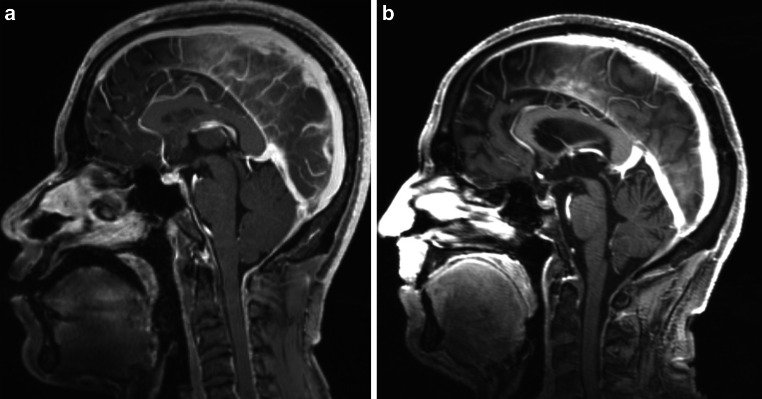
Sagittal contrast-enhanced MPRAGE (Magnetization Prepared-Rapid Gradient Echo) slices in a 34-year-old man with SIH due to a ventral dural tear at TH1/2 (a) and a 60-year-old man with an ocular myasthenia and a normal MRI (b). SVM-based SIH score is 2 in (a) and 0 in (b)
